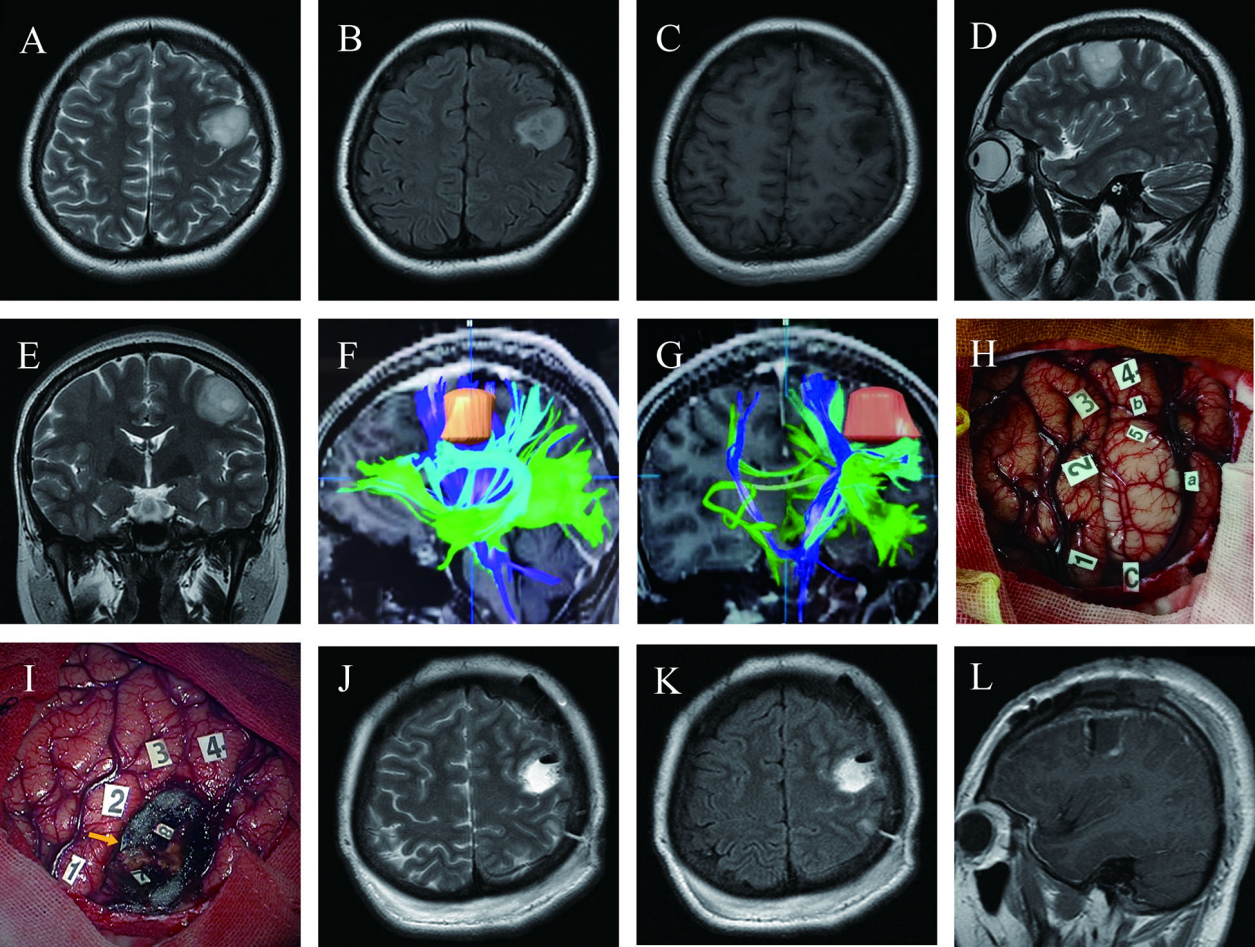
Maximum safe resection of oligodendroglioma harboring IDH mutation and 1P/19Q codeletion (WHO Grade 3) in the inferior left precentral gyrus under awake craniotomy in a 27-year-old woman presenting with seizures (Case 9); (A–E) Pre-operative T2 axial, T2 FLAIR axial, T1-weighted gadolinium-enhanced axial, T2 sagittal, and T2 coronal MRI revealed the tumor’s location precisely in the inferior lateral left hand knob of the precentral gyrus. (F, G) DTI-based reconstructed fibers showed arcuate fasciculus beneath the lesion and slightly distorted, and the pyramidal tract was medial to the lesion; (H) Intraoperative view before tumor resection; Tumor borders marked by letters (a, anterior; b, superior; c, inferior). Number of tags denotes positive DES mappings (1, paresthesia of the right little and ring fingers; 2, numbness of the right thumb; 3, paresthesia of the right corner of mouth; 4, motor responses in the right corner of mouth; tag 5 is on the surface of the tumor, where speech arrest and convulsions of the right corner of the mouth were induced during DES); (I) Intraoperative view after tumor resection based on the functional boundary; The tumor was removed until DES mapping encountered eloquent brain areas at cortical and subcortical levels (Tag 7, movement of the right hand during DES; 8, movement of the right corner of the mouth; 9 with a yellow arrow, an electrifying sensation of the right index finger); (J–L) Twenty-four hours postoperative T2 axial, T2 FLAIR axial, and T1-weighted gadolinium-enhanced sagittal MRI demonstrated total tumor resection.
